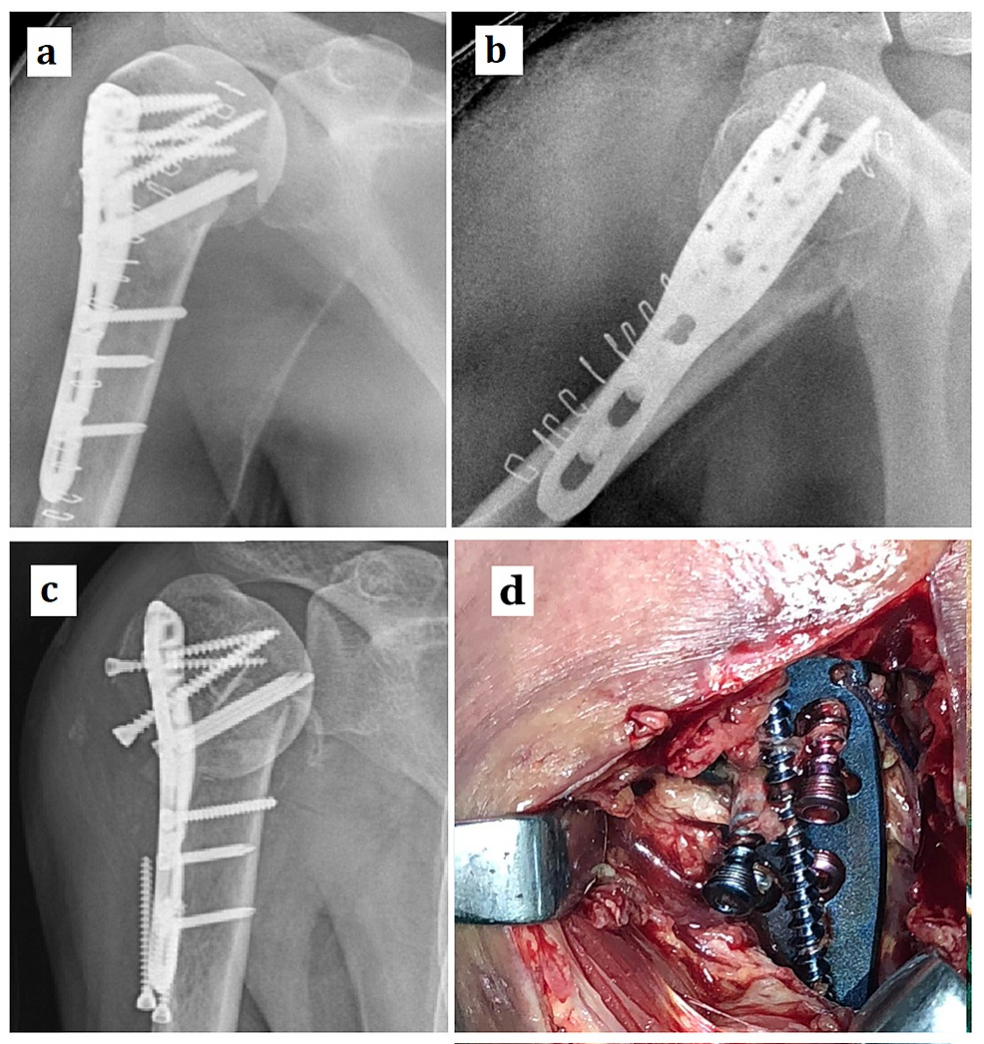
Postoperative radiographs, AP (a) and lateral (b), of a two-part proximal humerus fracture fixed with a proximal humerus locking plate have been shown. Following a seizure episode, the patient sustained a catastrophic fracture fixation failure with varus collapse of fracture (c). During the revision surgery, the intraoperative picture (d) shows the proximal locking cancellous screws migrated out of the proximal fragment. AP - anteroposterior
